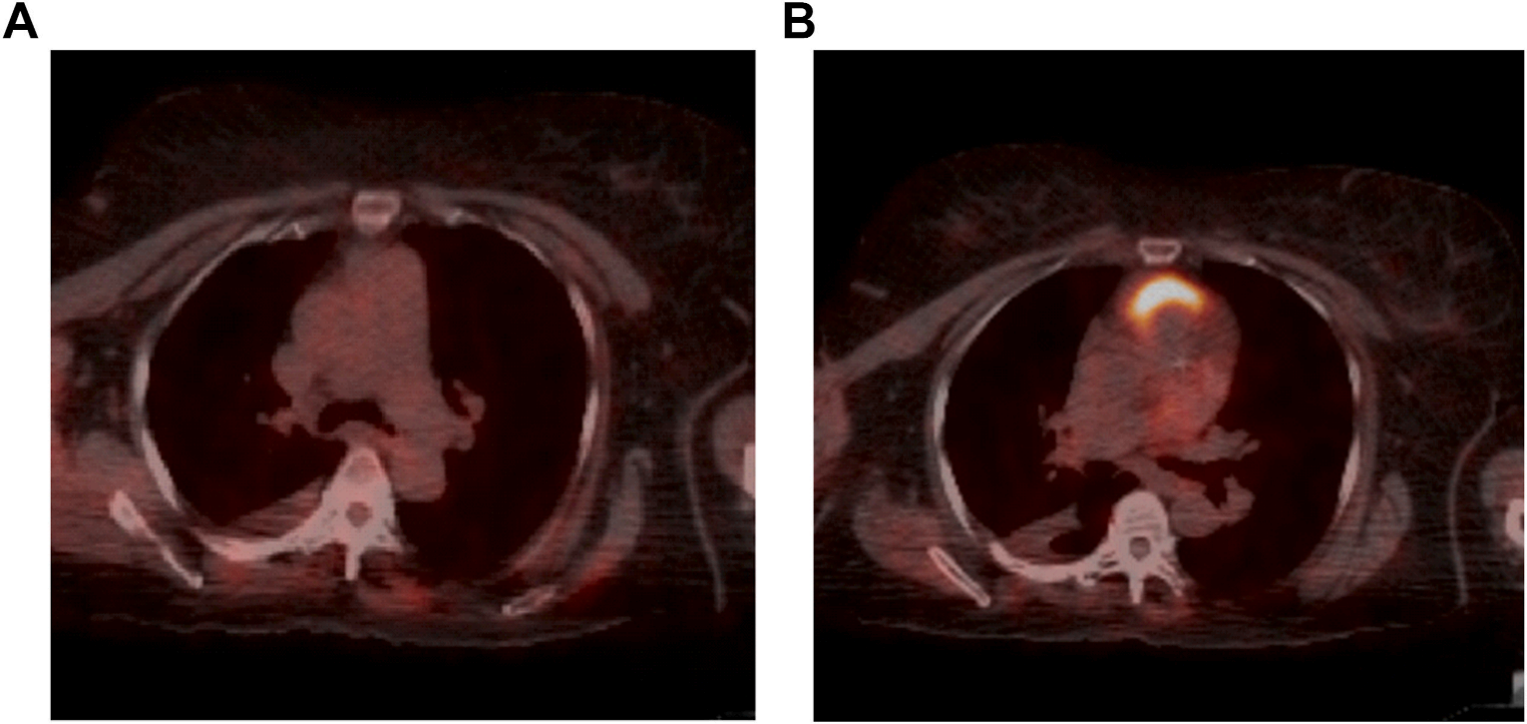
(A and B) Axial images of PET/CT scan which shows significant reduction of the PET activity pleural based masses.
